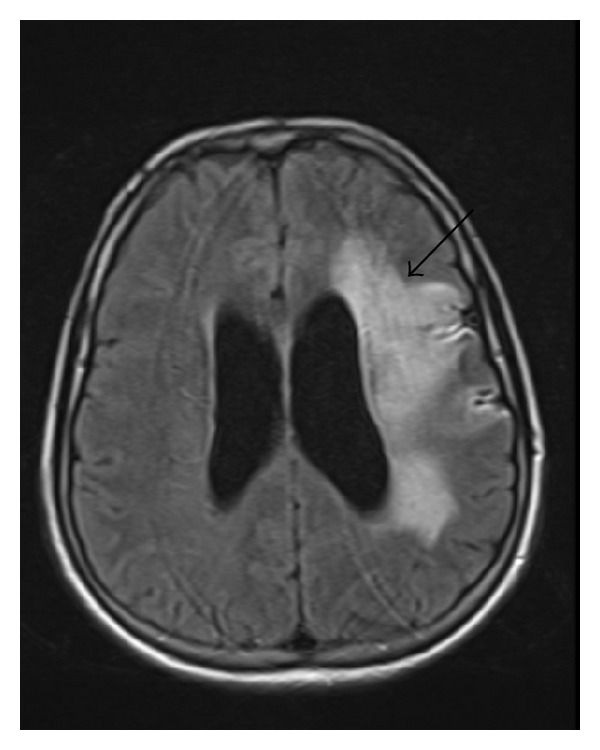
Infarct region compatible with large-size MCA infarct in left frontotemporoparietal lobes on cerebral MRI.
